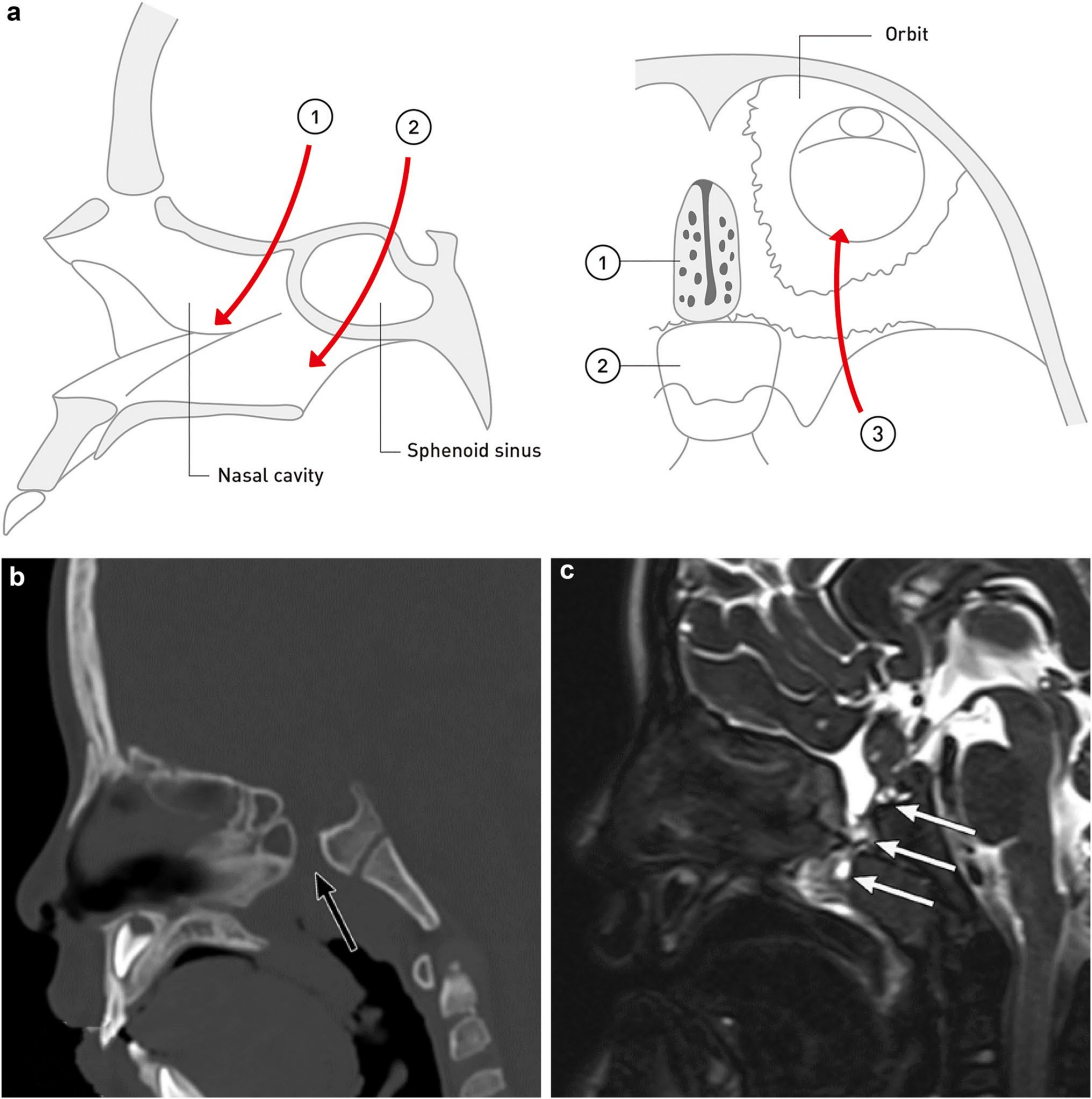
Congenital skull base cephalocele. a Classification of congenital skull base cephalocele. Skull base cephaloceles are classified into (i) transethmoidal, (ii) transsphenoidal, and (iii) spheno-orbital. The arrows indicate the pathway by which intracranial contents protrude into the extracranial space through a skull base defect. b Congenital skull base cephalocele (transsphenoidal cephalocele) in a 5-year-old boy with bacterial meningitis and growth hormone deficiency. Sagittal head computed tomography shows a partial defect of the sphenoid bone (black arrow). c Sagittal T2-weighted magnetic resonance image shows herniated contents extended into the nasopharynx through the defect of the sphenoid bone (transsphenoidal cephalocele). Herniated content shows heterogenous intensity, with fluid components showing high intensity, and herniated brain parenchyma and dysplastic gliotic tissue showing iso intensity (white arrows). The pituitary gland has a deformity and is herniating into the sphenoid bone defect
